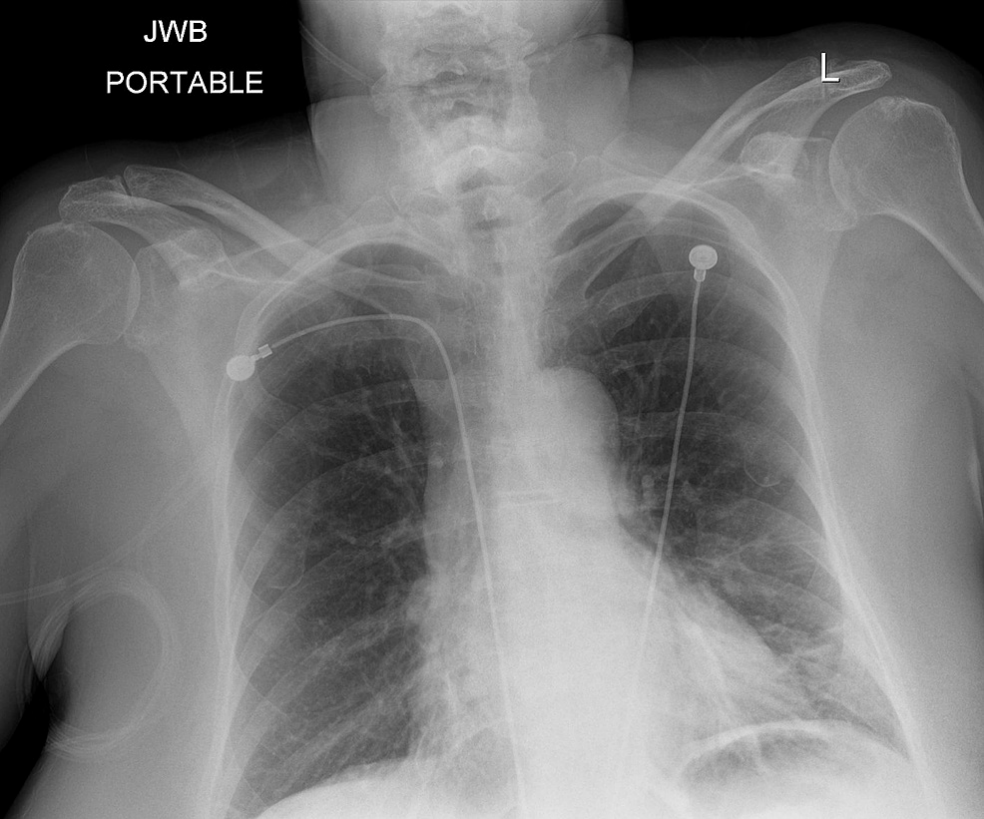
Post-bronchoscopy chest X-ray
